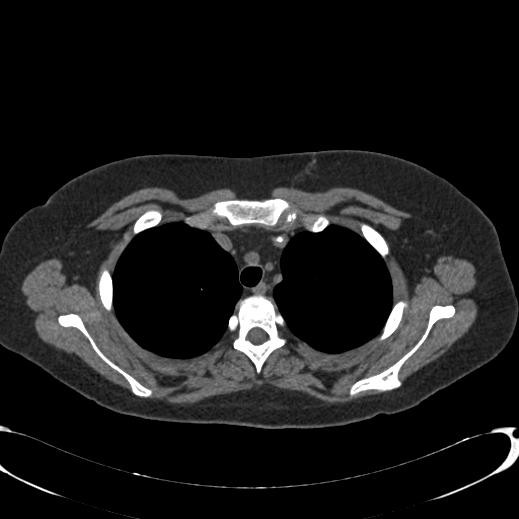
Transverse plane computed tomography image of the chest. An area of visible density in the left innominate vein as depicted on the transverse plane
